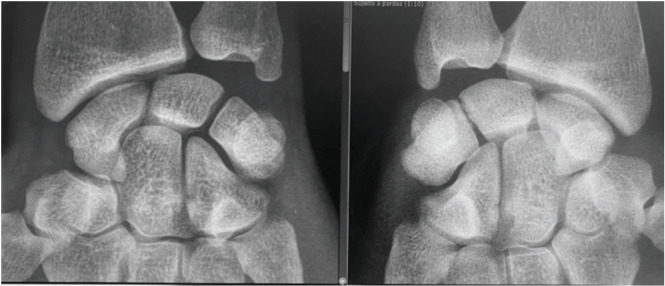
Plain comparative radiograph showing congenital Luno-triquetral synostosis on the right.
